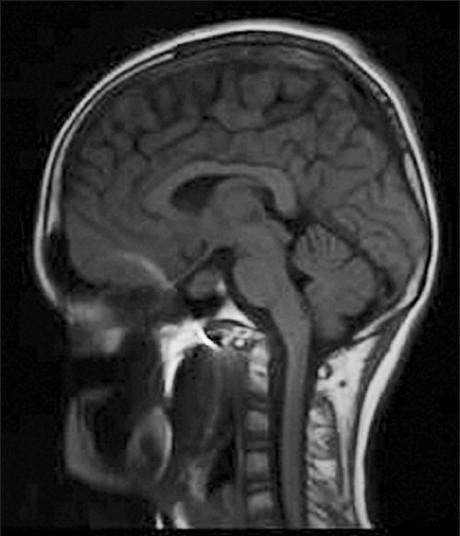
T1-weighted sagittal MRI revealing a pituitary tumor with minimal suprasellar extension in a child
